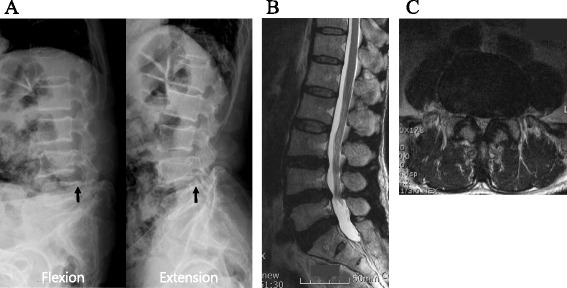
Preoperative radiographs and lumbar magnetic resonance imaging. a Dynamic plain radiographs showing degenerative spondylolisthesis of lumbar vertebral body 4 on lumbar vertebral body 5. The radiographic finding showed anterior slipping of lumbar vertebral body 4 on lumbar vertebral body 5 when the patient bent his back (*arrow*). An extension view shows the slight reduction of anterior slippage of lumbar vertebral body 4 on lumbar vertebral body 5 (*arrow*). b Sagittal view showing disc protrusion and stenosis of both foramen on lumbar vertebral body 4 and lumbar vertebral body 5. The dural sac was compressed ventrally and dorsally at lumbar vertebral body 4 and lumbar vertebral body 5 on a sagittal view of T2-weighted images. c Axial view showing disc protrusion, stenosis, and facet hypertrophy on lumbar vertebral body 4 and lumbar vertebral body 5. Narrowing of the spinal canal was found with both disc protrusion and ligamentum flavum hypertrophy
